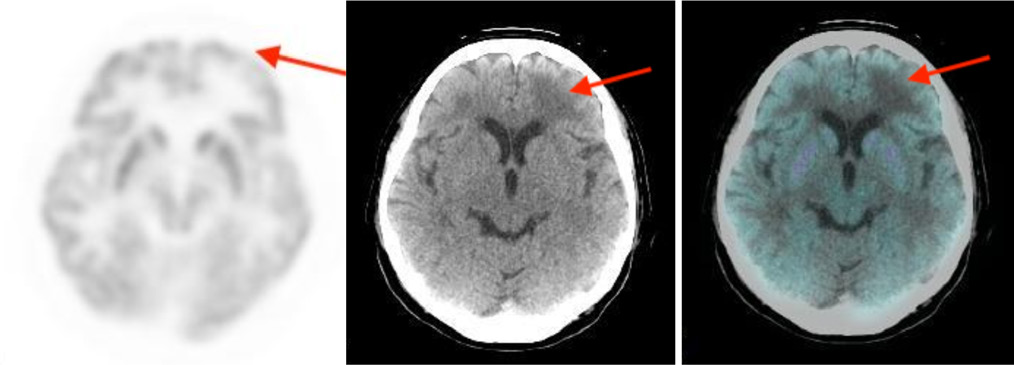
FDG PET (left) showed asymmetrical hypometabolism in left frontal lobe (red arrow) associated with peri-focal oedema on CT (middle).
